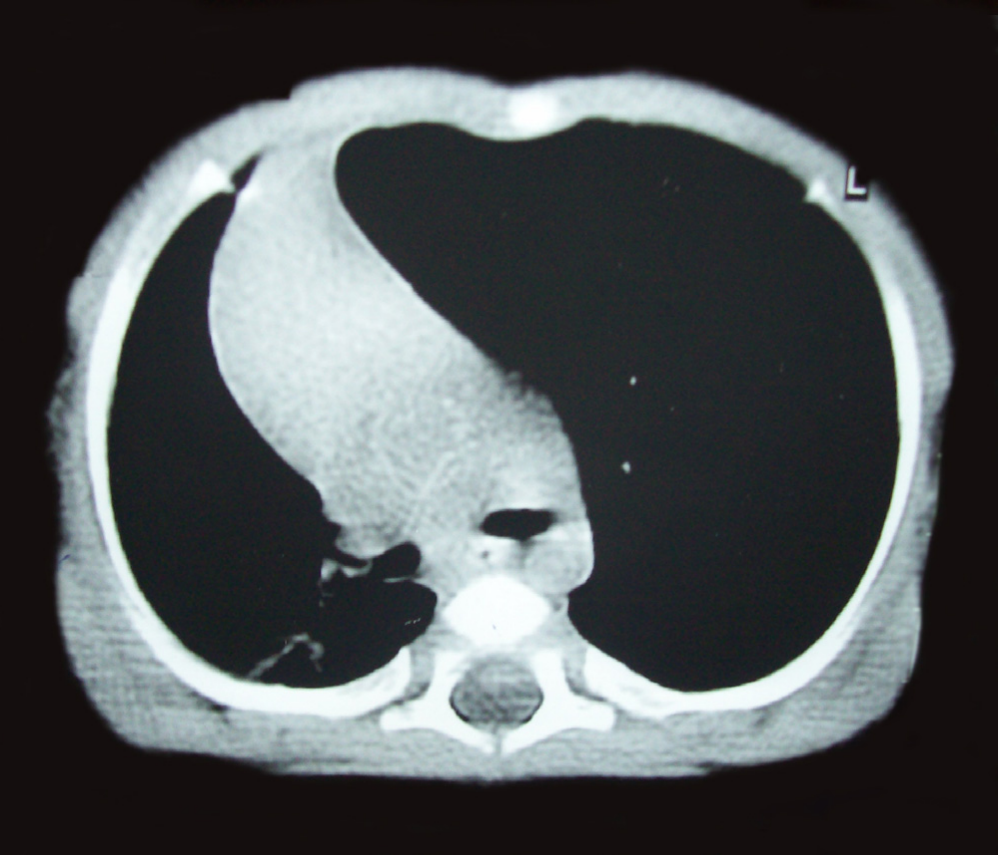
CT scan showed a hyperluscent, hyper extended lobe with midline substantial herniation and compression of the remaining lung. The mediastinum is significantly shifted away from the side of the abnormal lobe.
